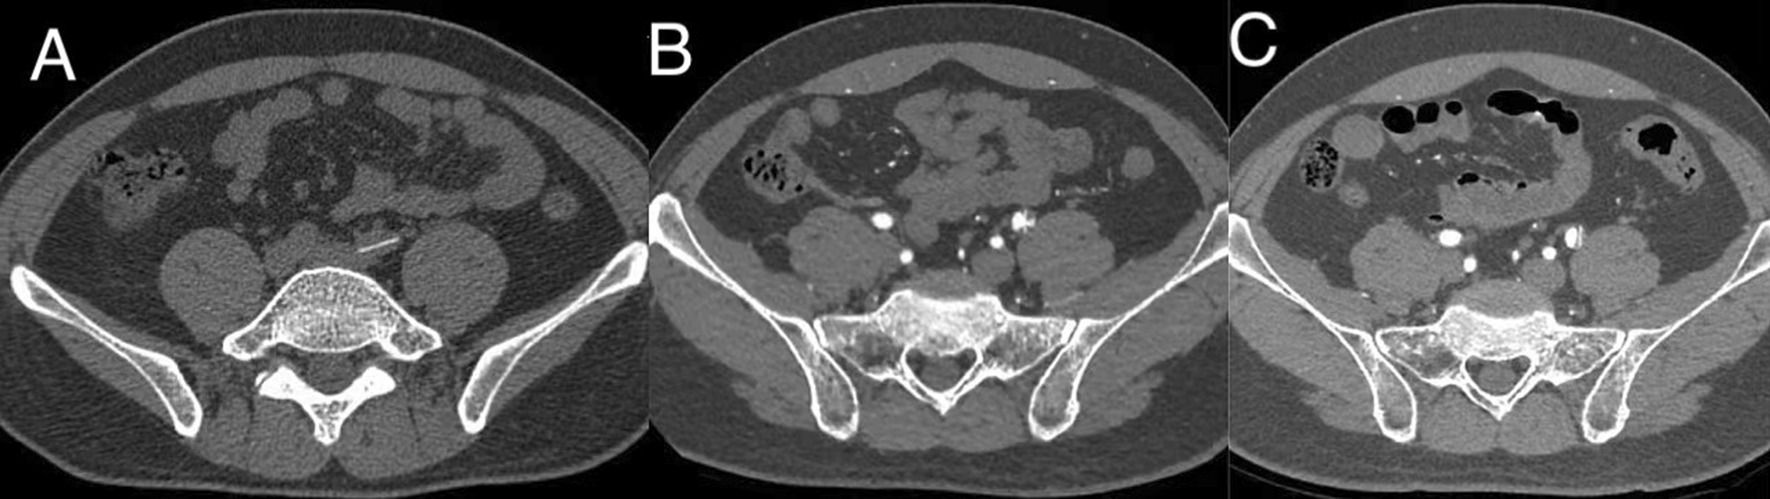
CT images of the aortic valve leaflet displacement over 10 years. A In 2010, the valve leaflet was located in the left common iliac artery; B In 2016, the valve leaflet was located in the left external iliac artery, and the stenosis at the embolization site was approximately 40%; C In 2020, the valve leaflet was embolized in the left external iliac artery, and the stenosis at the embolization site was approximately 30%. CT: Computerized tomography
